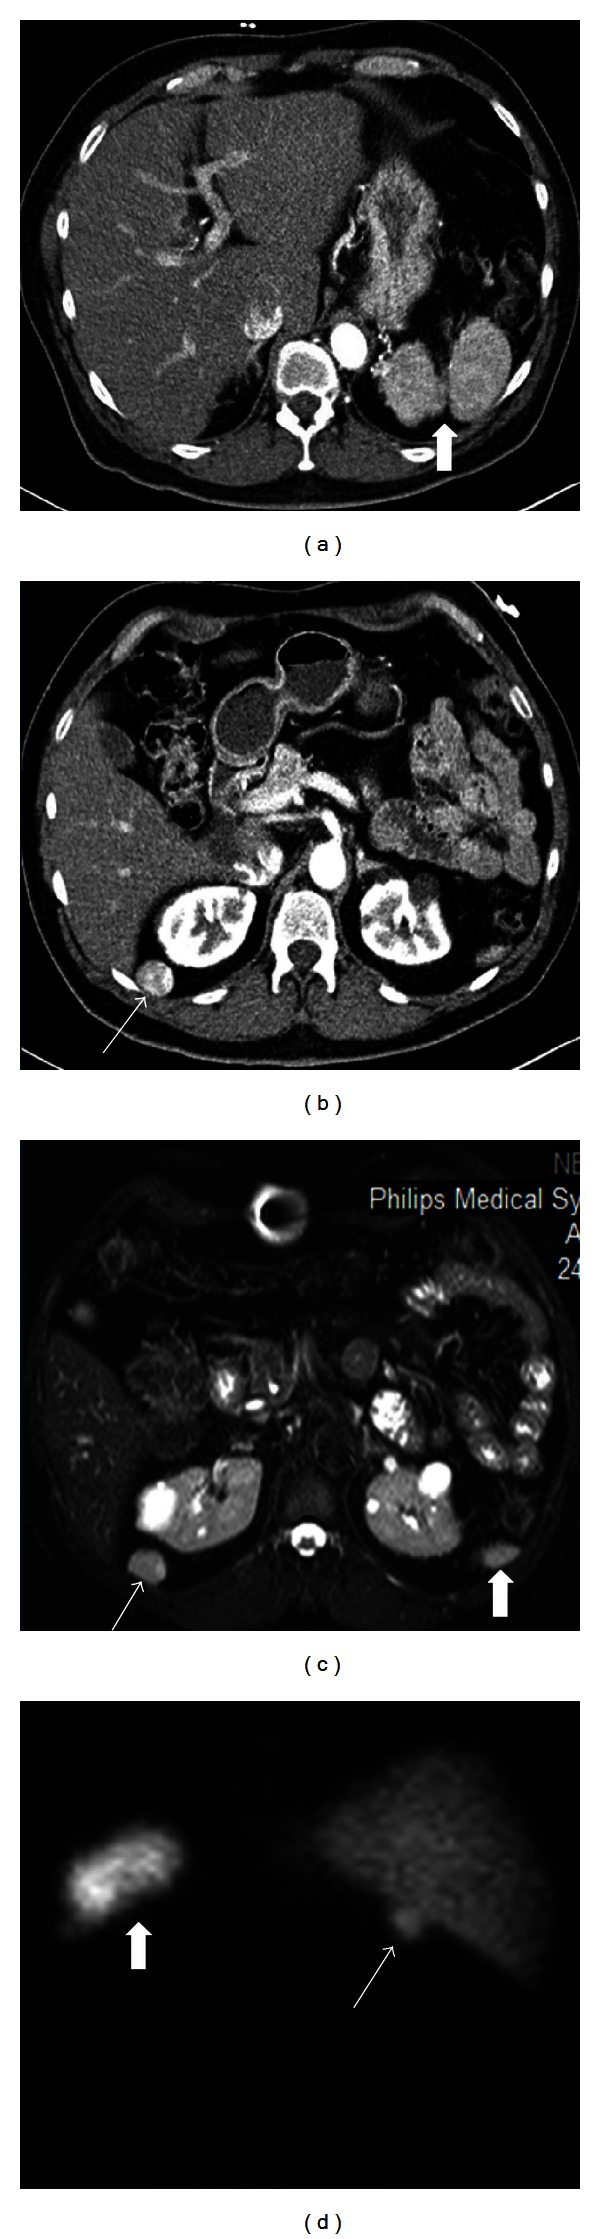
Splenosis in a 62-year-old male patient with a history of splenic trauma and splenectomy. Axial contrast enhanced CT images (a, b) show two splenic fragments (thick arrow) on the left upper quadrant (a) and a nodular mass (arrow) with a heterogenous contrast enhancement pattern as splenic tissue posterior to the liver and kidney (b). Axial T2 weighted image (c) demonstrates similar signal intensity of both the nodular mass (arrow) and inferior pole of the splenic fragments (thick arrow). Technetium-99 m posterior scintigraphy image (d) also shows uptake of the nodular mass (arrow) as splenic tissues on the left (thick arrow).
